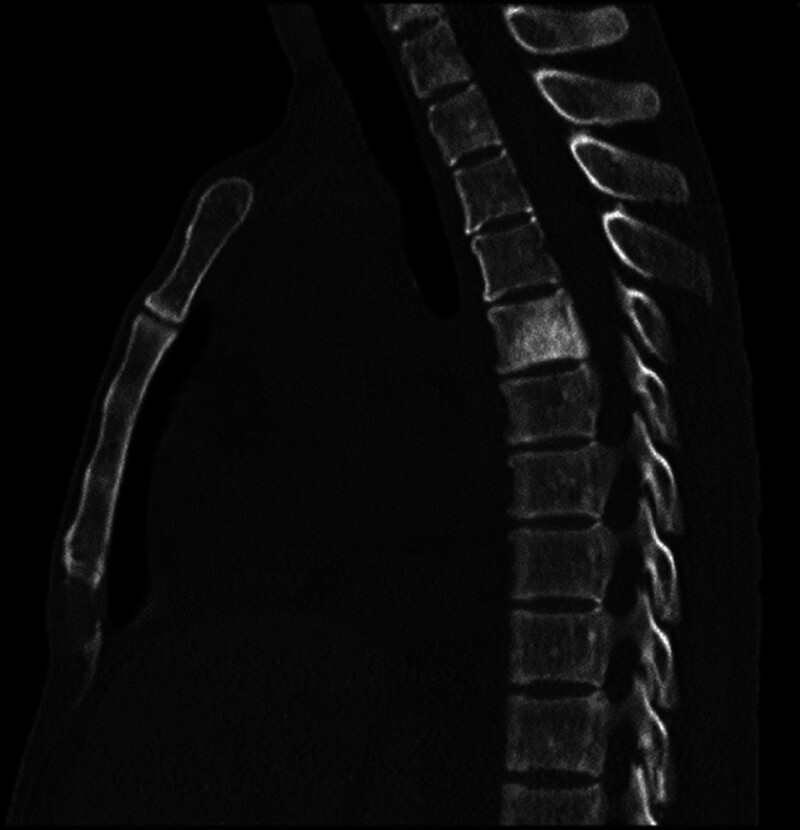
CT revealed the manifestations of bone sclerosis disease. CT = computed tomography.
